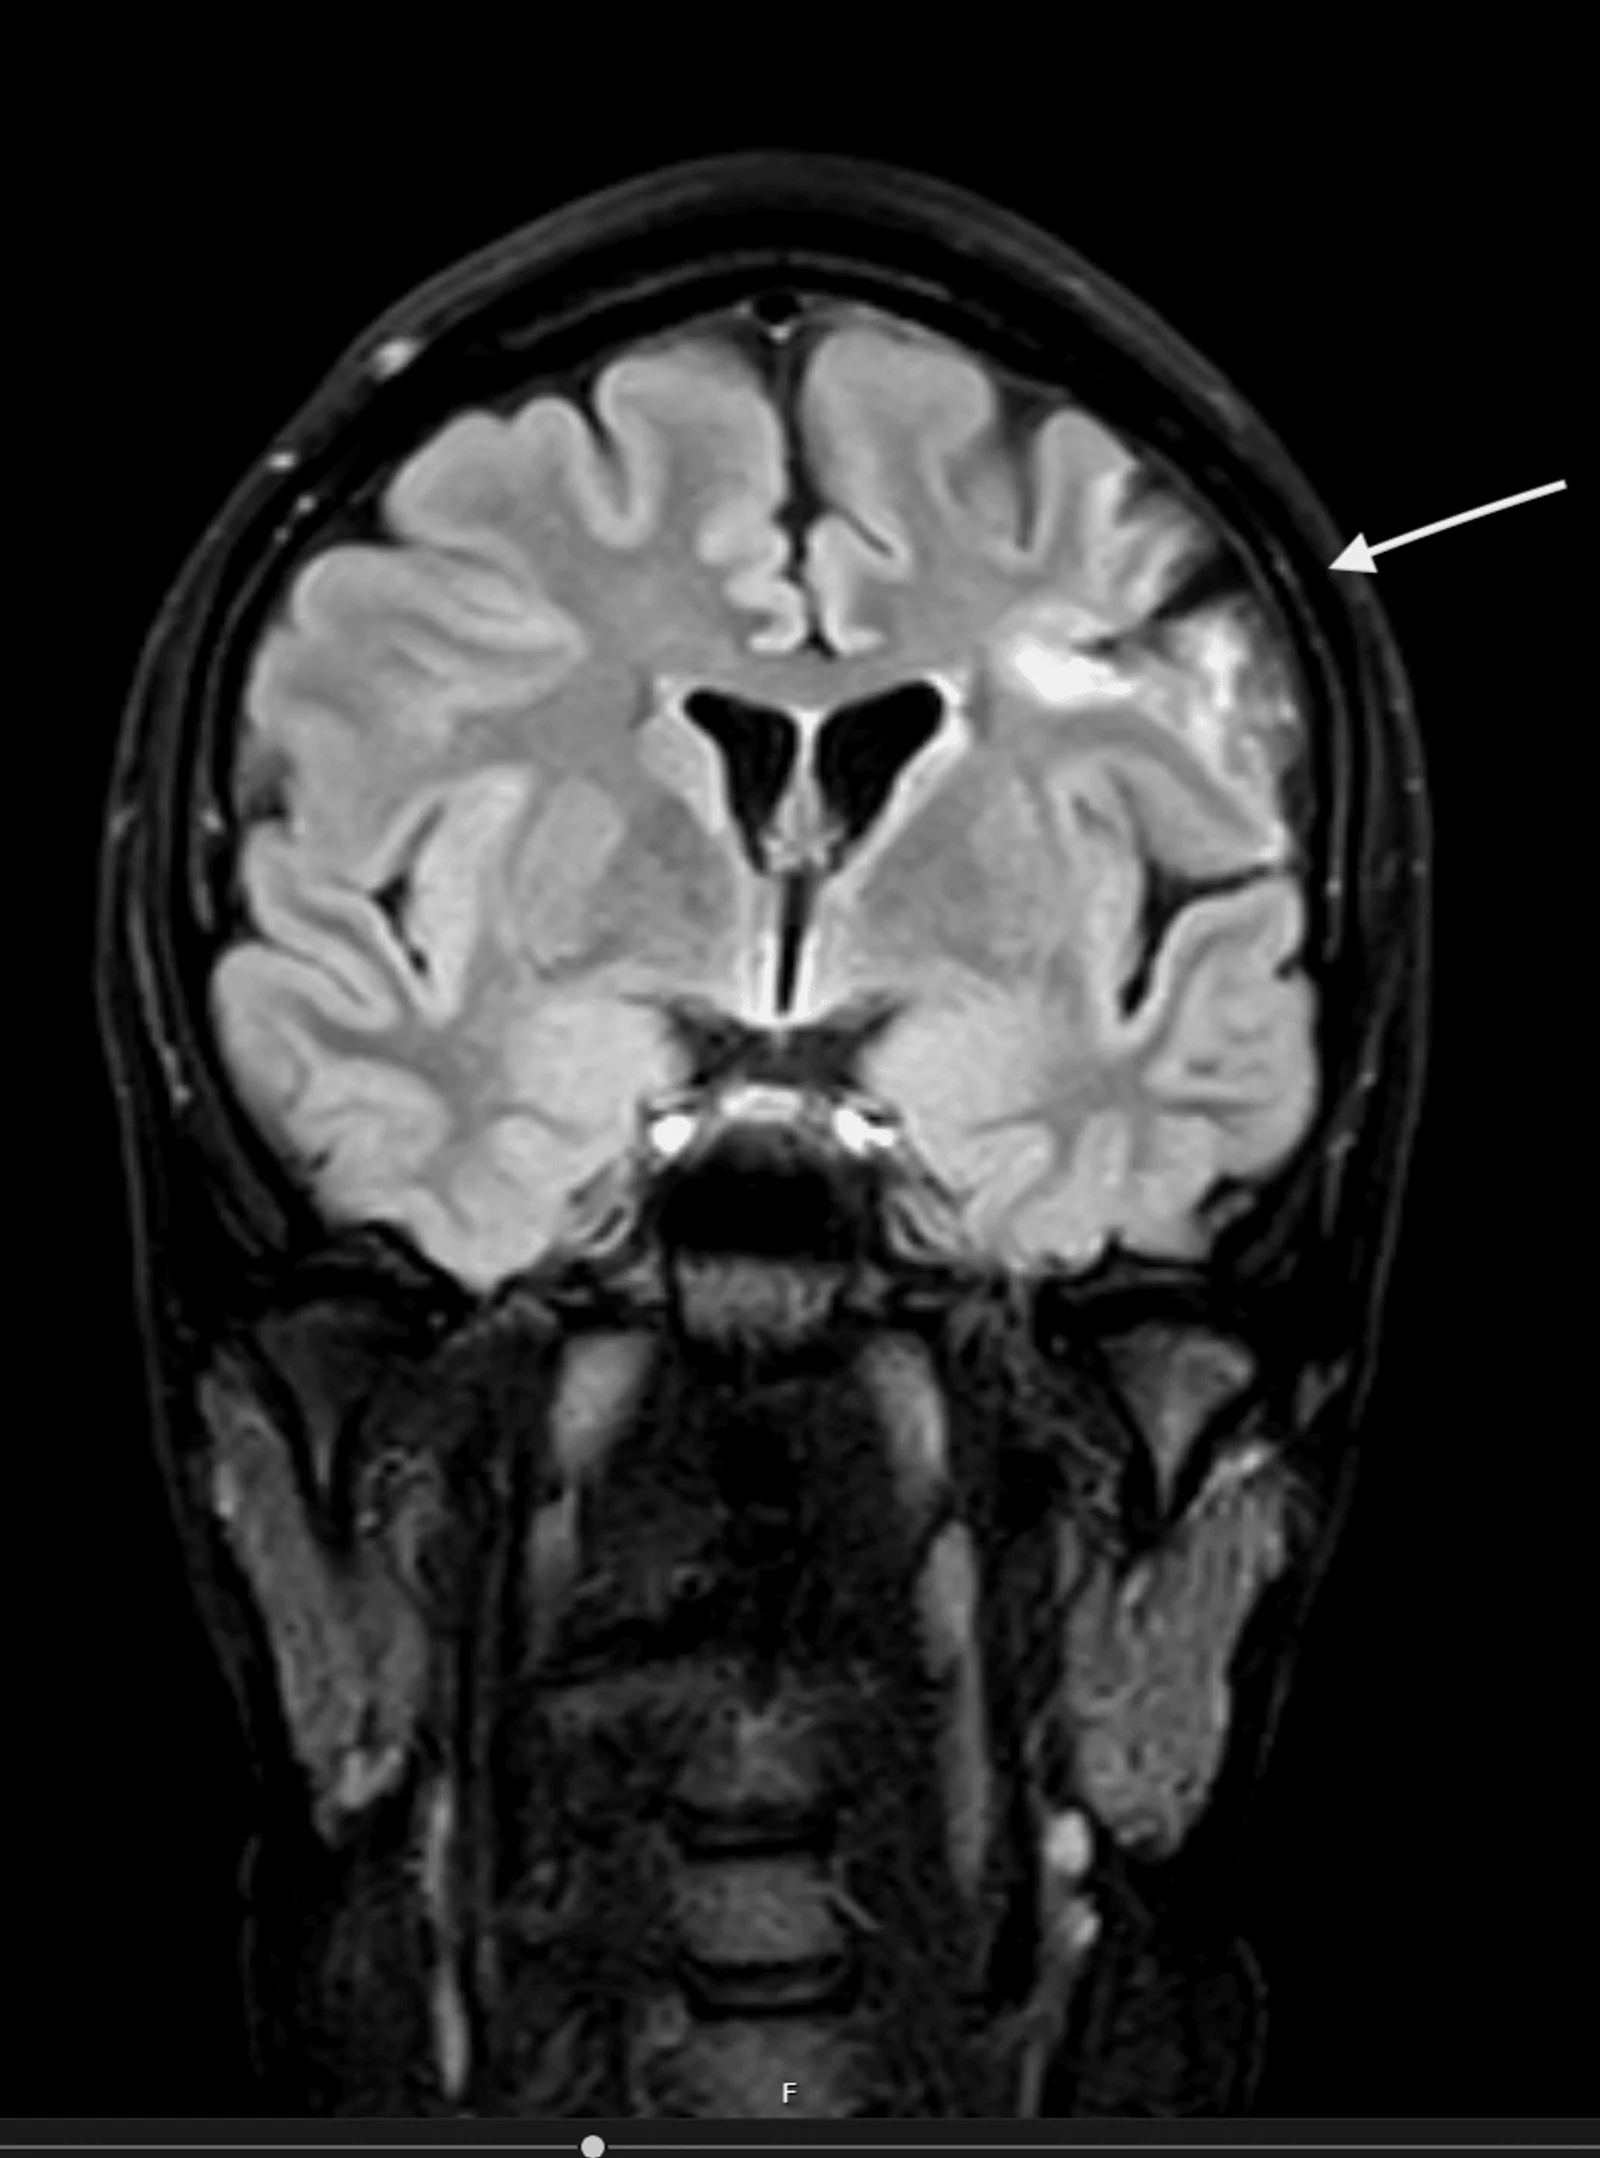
Coronal fluid-attenuated inversion recovery (FLAIR) MRI of the brain demonstrating cortical and subcortical hyperintensity in the left middle cerebral artery territory (arrow), consistent with an established infarction.
